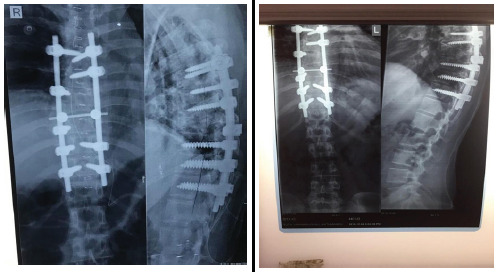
A) Post-operative 2^nd^ month, thoracic spine X-ray (anteroposterior and lateral view). B) Post-operative 6th month, anteroposterior and lateral view radiographs of thoracic spine.
